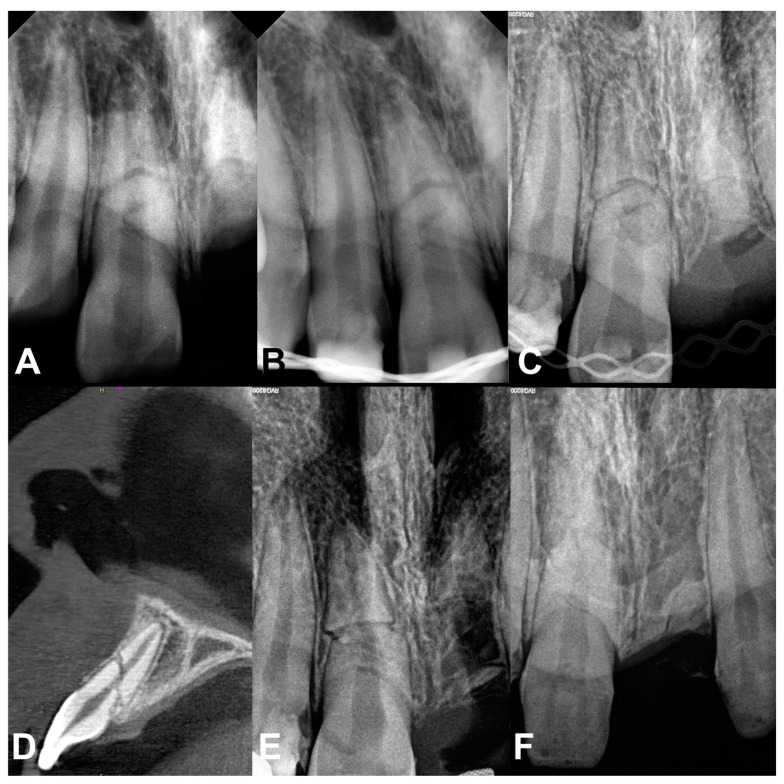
Dental radiographs and CBCT: (A) Radiograph after the trauma; (B) radiograph 7 days after trauma; (C) radiograph 3 months after trauma, teeth splinted with TTS; (D)cone beam computed tomography 6 months after trauma; (E) radiograph 2 years after trauma; (F) radiograph 5 years after trauma.
